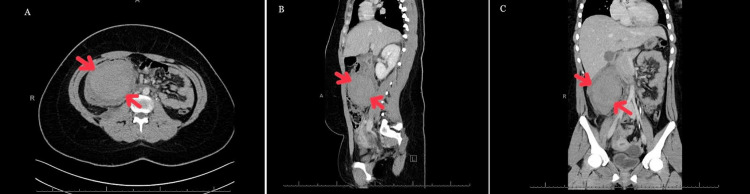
Abdominal contrast-enhanced CT images (A) Axial view revealed a hyperdense mass in the ascending colon (white arrow). (B) Sagittal view showing a hematoma at the ascending colon (white arrow). (C) Frontal view
